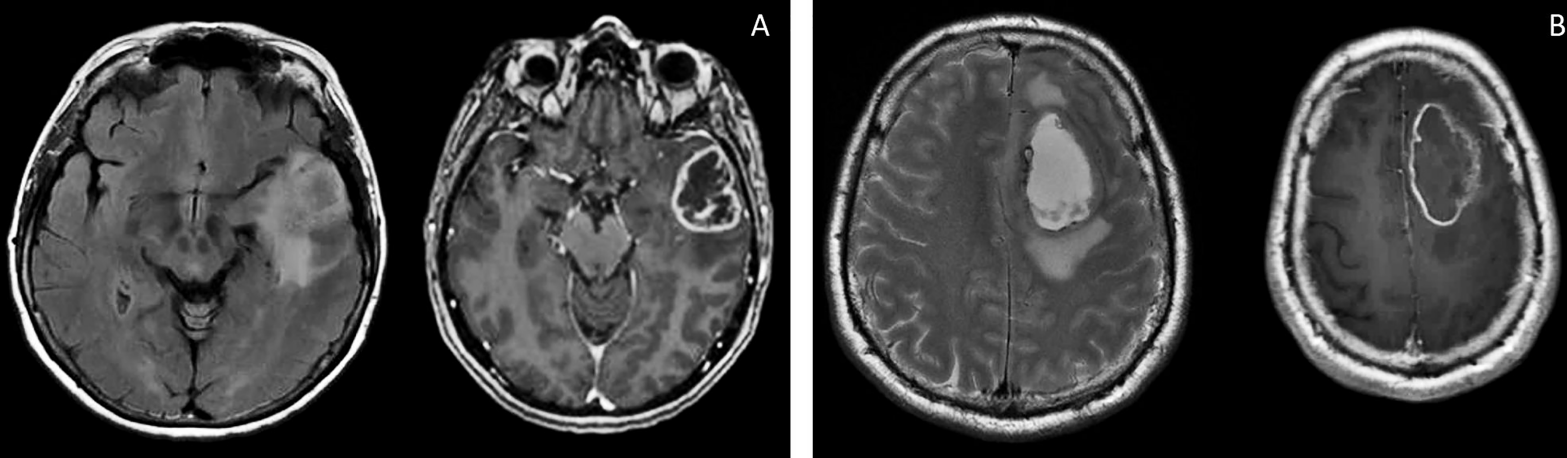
Brain MRI, T2 FLAIR, and T1 images of patients S (A) and G (B). There are the same typical heterogenic changes of the signal with a ring-like contrast enhancement and a signal typical for edema around the tumors.
